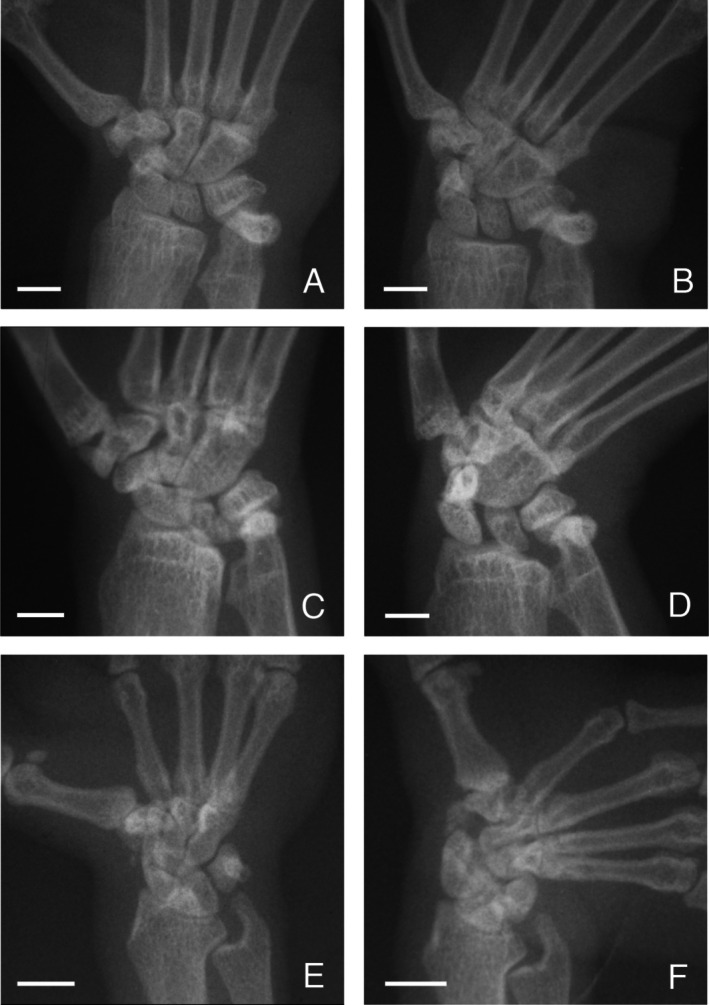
Radiographs of the hand in neutral position (left) and maximum ulnar deviation position (right) in a pronograde quadruped (
